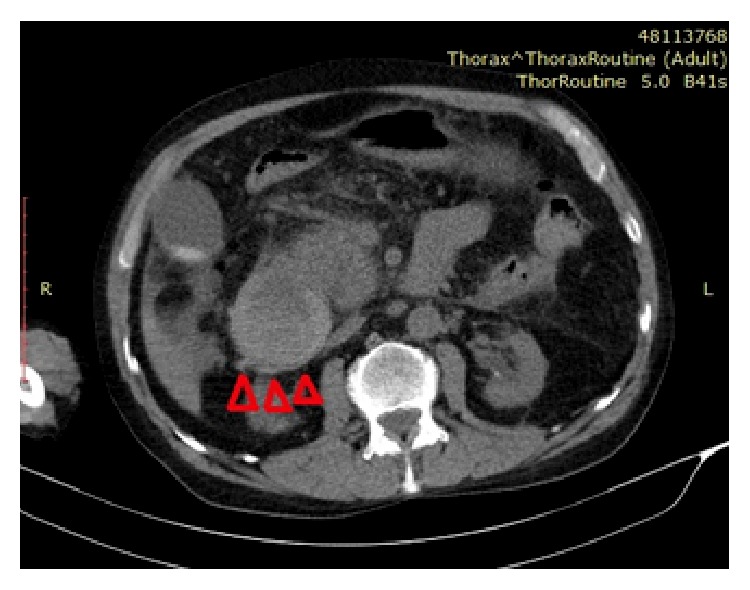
Computerized tomography image from the same axial plane as magnetic resonance imaging (Figure 6), showing intramural hematoma (red arrow heads).
